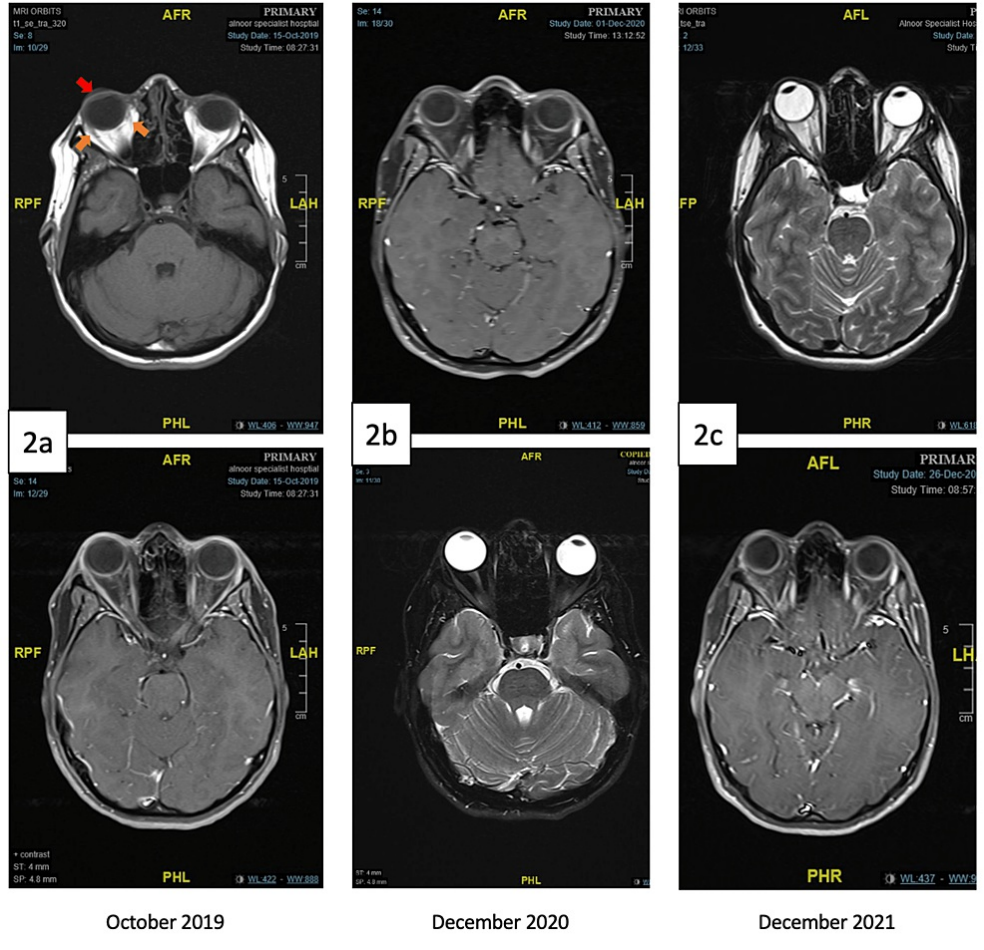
MRI of the orbit: a: (October 2019) significant proptosis of the right eye globe (red arrow) and swelling and enhancement of the extra-conal soft tissues (orange arrows), b: (December 2020) resolution of the proptosis and extra-conal findings post three cycles of rituximab therapy, c: (December 2021) sustained radiologic remission of the idiopathic orbital inflammation one-year post rituximab therapy.
